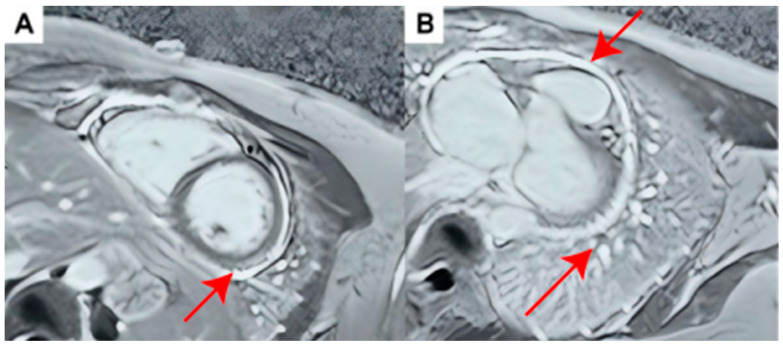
Cardiac Magnetic Resonance Imaging Findings. CMR was performed approximately 2 weeks after the most recent symptomatic flare, when her symptoms had improved, and CRP was within the normal range. Short-axis late gadolinium enhancement images. (A) Subepicardial delayed enhancement (red arrow) of the inferior and lateral walls of the left ventricle; (B) Extensive pericardial thickening with delayed enhancement (red arrow).
